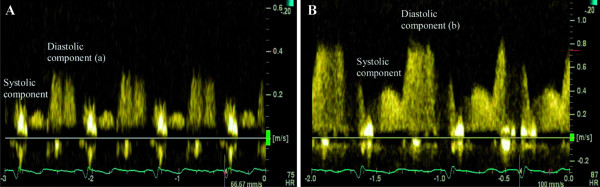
Coronary flow by spectral Doppler. The greater component during diastole and the smaller component during systole at rest (A) and during hyperaemia (B). CFR = b/a. CFR > 2 (an example of normal CFR).
